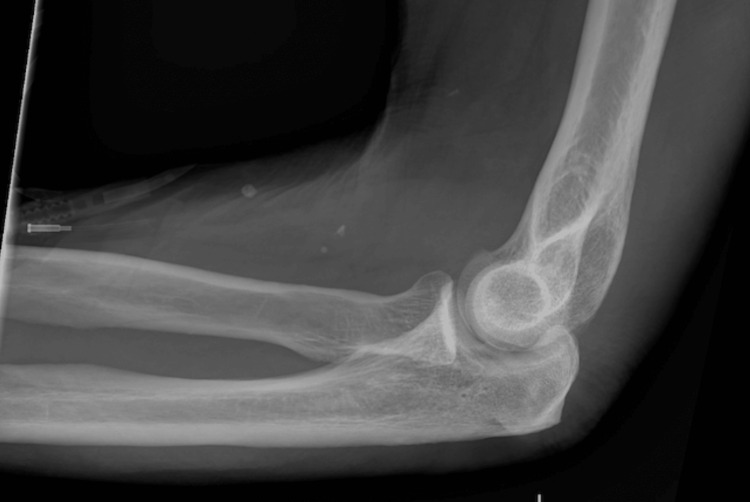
X-ray of the left forearm Negative for any fractures
